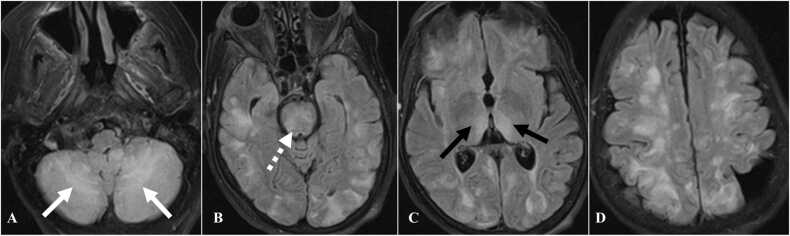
Immune mediated toxic encephalopathy in a 69-year-old woman with lung cancer on Pembrolizumab therapy. A to D. Axial FLAIR images of MRI of the brain demonstrate subcortical and periventricular hyperintensities. Also seen are hyperintense areas in the cerebellum (white arrow), pons (dashed white arrow), and thalamus (black arrows).
